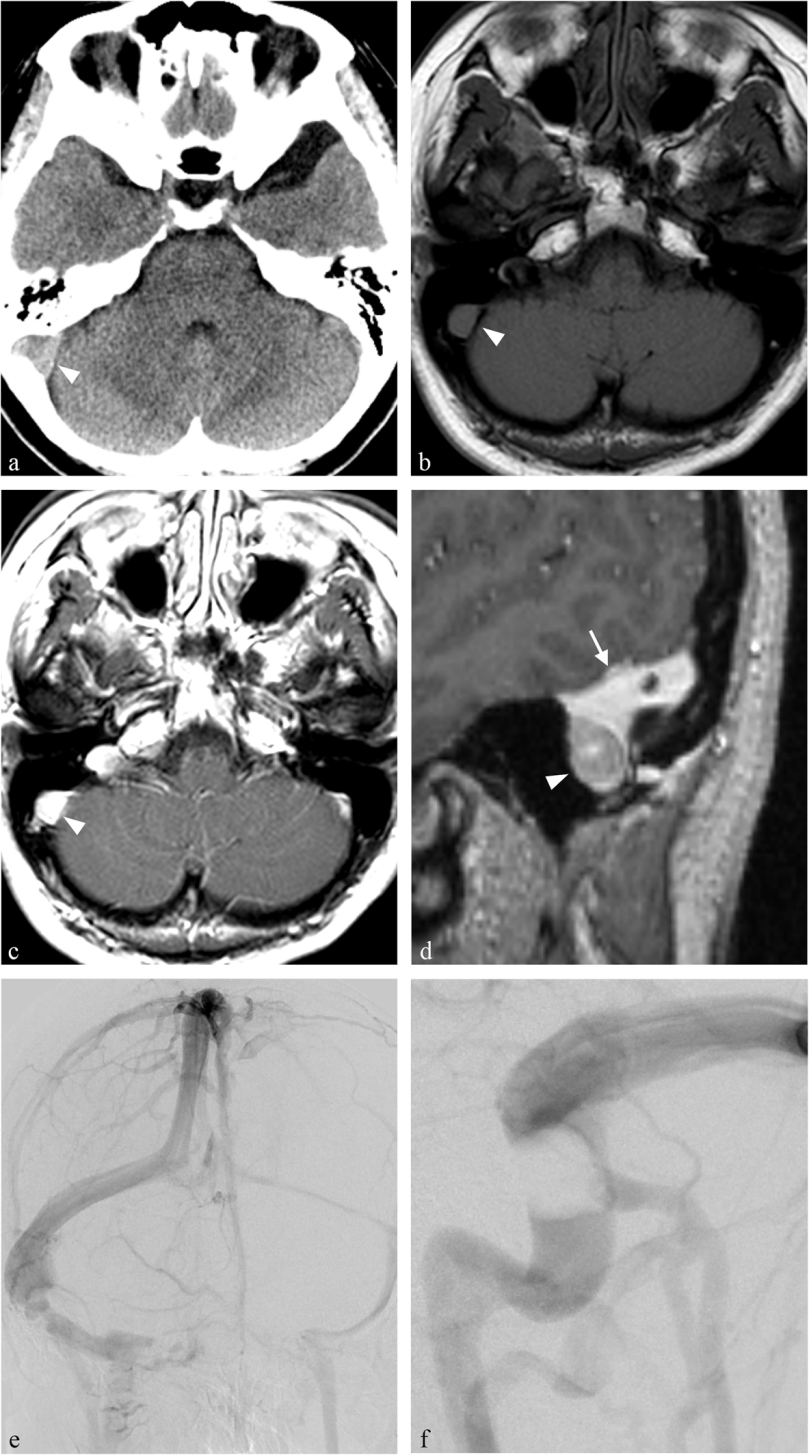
a Preoperative computed tomography (CT) scan revealing no intracranial space-occupying lesions or hydrocephalus except an asymptomatic arachnoid cyst of the left middle fossa. b–c Preoperative magnetic resonance images showing the lesion in the right sigmoid sinus (*arrowhead*) appearing as isointense on T1-weighted image (b), and with homogeneous enhancement following intravenous administration of gadolinium (c). No obvious dural tail sign is present. d Sagittal gadolinium-enhanced T1-weighted images revealing the mass lesion (*arrowhead*) located under the transverse sinus (*arrow*). Mass lesion causes severe luminal narrowing. e Conventional angiogram demonstrating the dominant right transverse sinus with hypoplastic left transverse sinus, and subtotal occlusion of the dominant right sigmoid sinus in the venous phase, with antegrade right transverse flow and poor collateral flow indicating this flow is dependent even in subtotal occlusion. f Conventional angiogram, venous phase, showing the mass lesion apparently located at the inner sinus wall mimicking venous thrombosis
